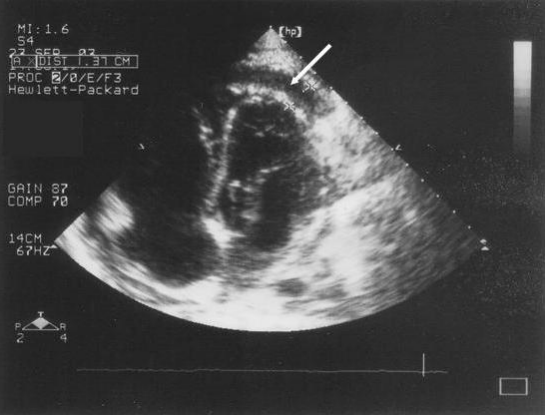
Echocardiogram showing apical collection of fluid in the pericardial space (white arrow).
